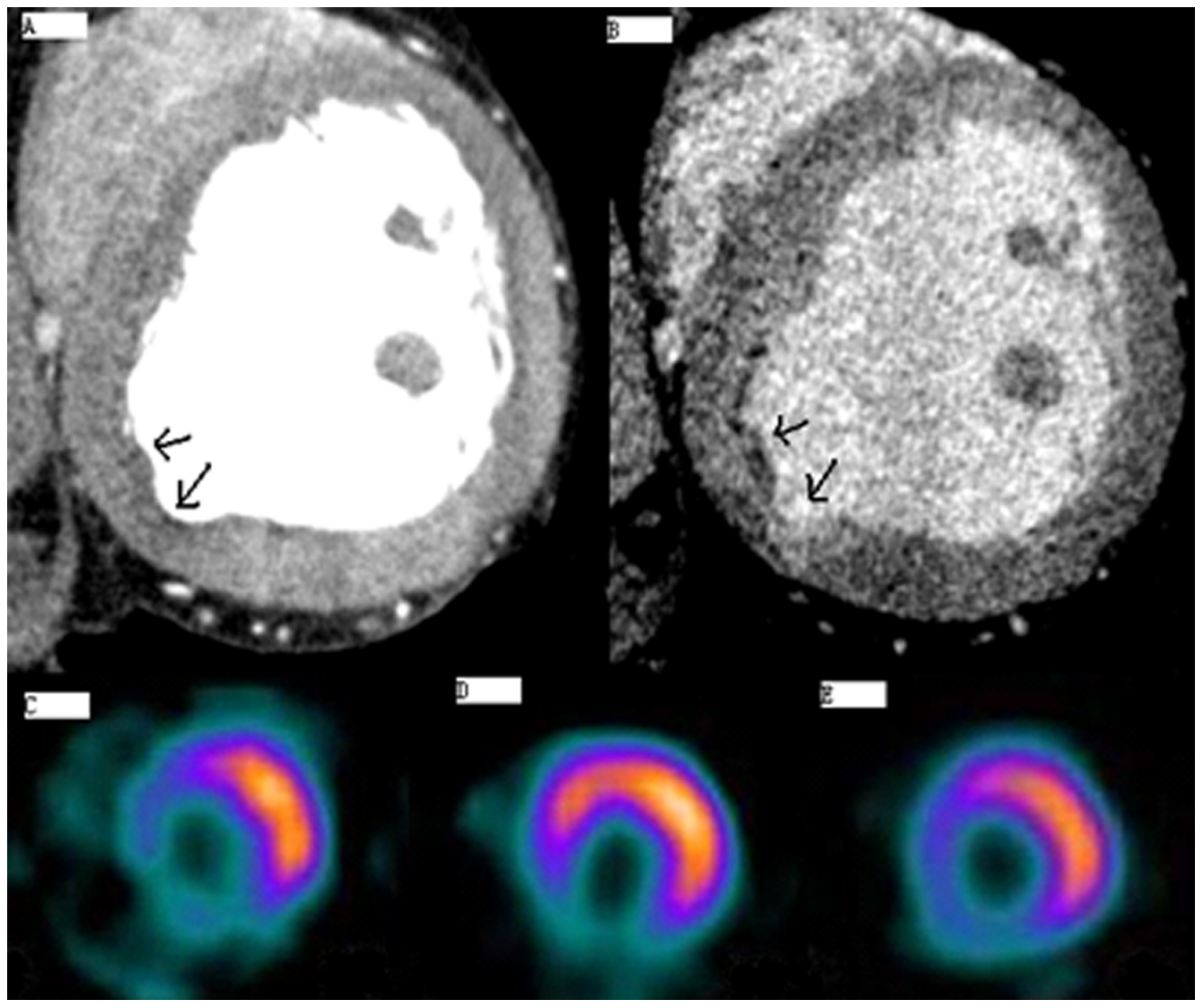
Representative Myocardial Perfusion Images of the Left Anterior Descending Coronary Artery (LAD) and Right Coronary Artery (RCA) using both MSCT and SPECT Examination. 3A: MSCT early scanning; 3B: MSCT late scanning; 3C: Stressed thallium-201 (^201^TI)-SPECT imaging; 3D: ^99m^Tc-MIBI imaging; 3E: ^201^TI-SPECT late resting image.
